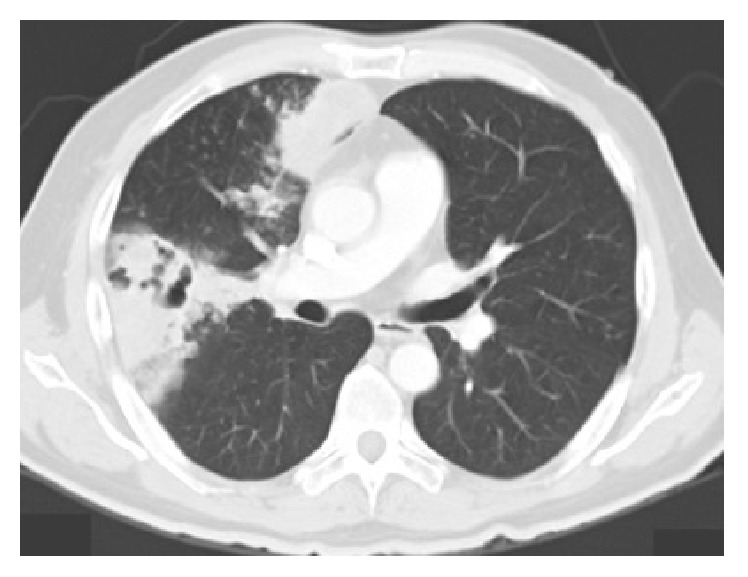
Axial computed tomography showing middle right lobe involvement.
